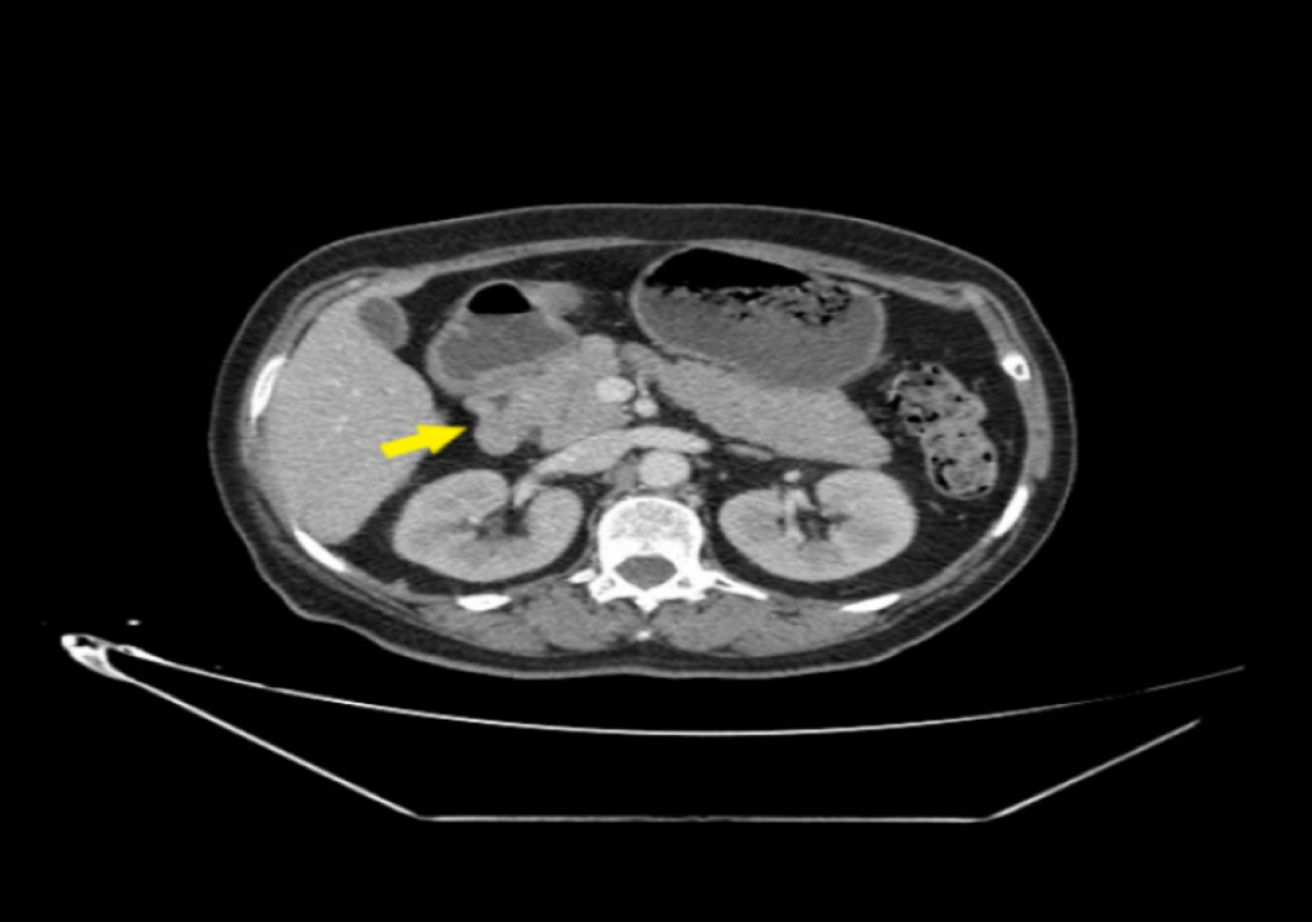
Abdominal computed tomography showing Grade B acute pancreatitis: head of pancreas
